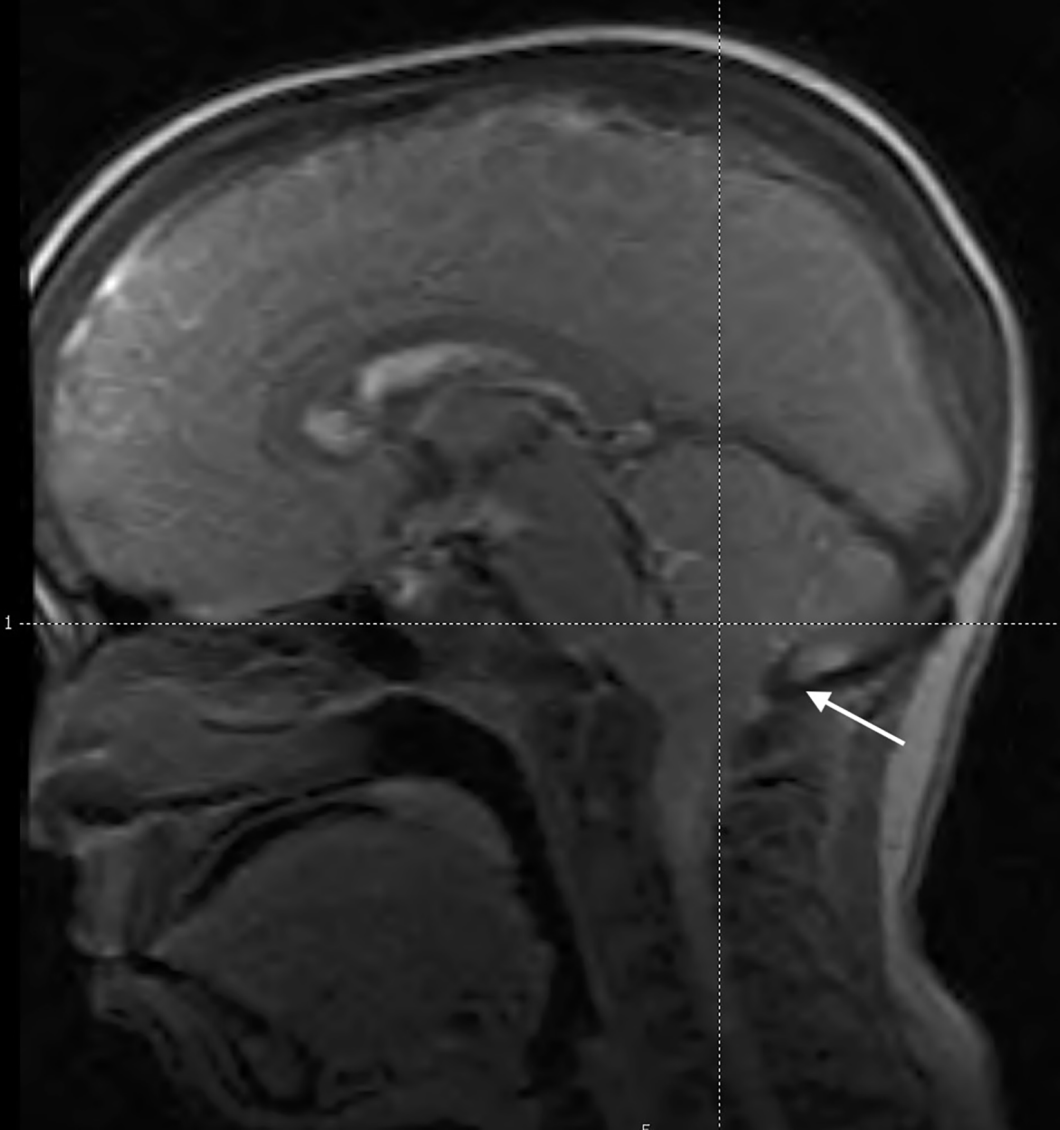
Midsagittal magnetic resonance image of the head in an 11.5-year-old male patient with XLH showing caudal descent of the cerebellar tonsils through the foramen magnum (Arnold-Chiari 1 malformation) (white arrow). He reported transient headache. Scaphocephaly was also evident.
